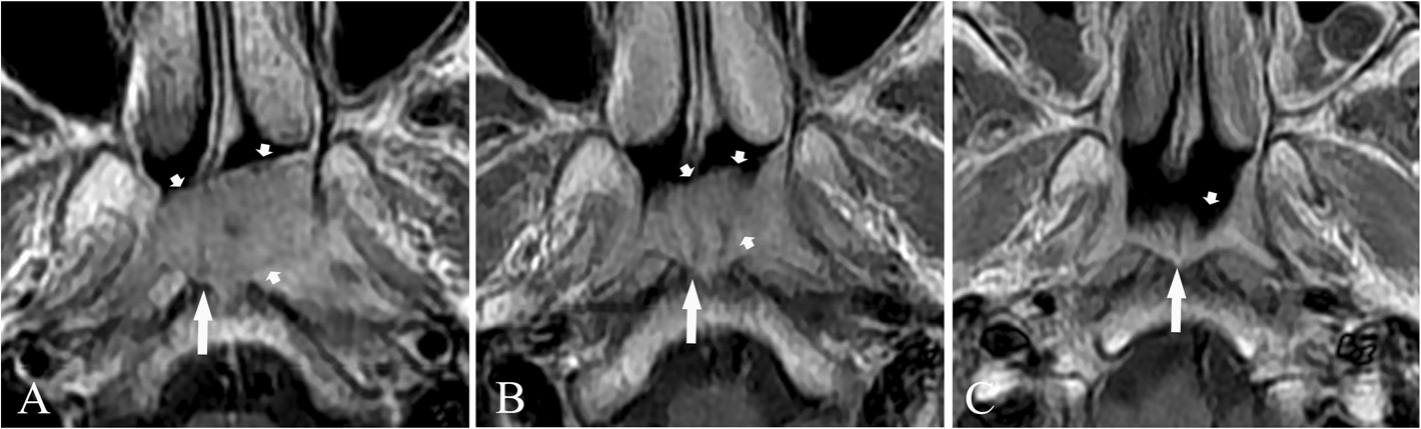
Treatment response, as determined by axial T1-weighted contrast-enhanced magnetic resonance imaging (MRI), in a 12-year-old nasopharyngeal carcinoma (NPC) patient with a small tumor coexisting with hypertrophic adenoids. A, before treatment, the NPC originated in the roof of the nasopharynx (short arrows) and could not be clearly distinguished from hypertrophic adenoids (long arrow). B, 10 days after the end of neoadjuvant chemotherapy, a partial response (PR) was achieved for the tumor (short arrows), and stripes (long arrow) became visible in hypertrophic adenoids. C, 3 months after the end of radiotherapy, a complete response (CR) was achieved for the tumor, a PR was demonstrated for hypertrophic adenoids, and the stripes on hypertrophic adenoids became more visible (long arrow). Note that hypertrophic adenoids were asymmetric (short arrow) most likely because of previous tumor invasion.
